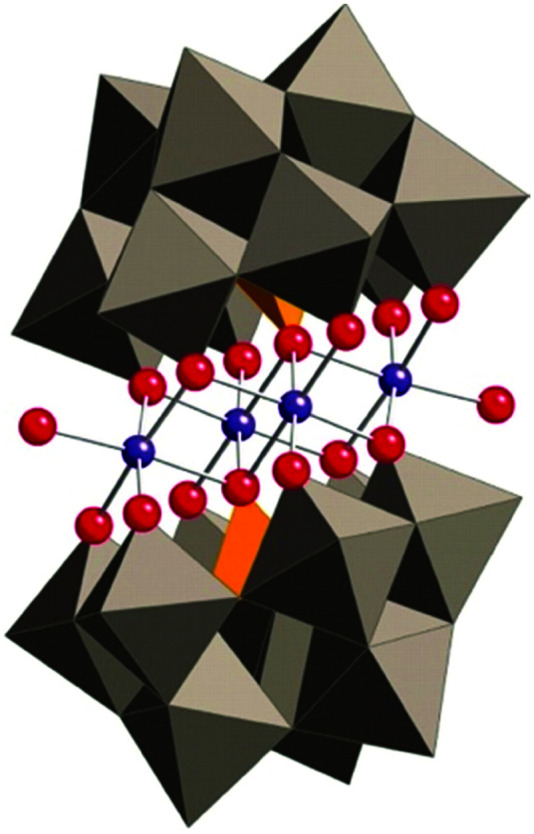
X-Ray structure of Na_10_[Co_4_(H_2_O)_2_(PW_9_O_34_)_2_] in combined polyhedral ([PW_9_O_34_] ligands) and ball-and-stick (Co_4_O_16_ core) notation. Co atoms are purple; O/OH_2_(terminal), red; PO_4_, orange tetrahedra; and WO_6_, gray octahedra. Hydrogen atoms, water molecules, and sodium cations are omitted for clarity. Reprinted with permission from ref. 1583 Copyright 2010. AAAS.
